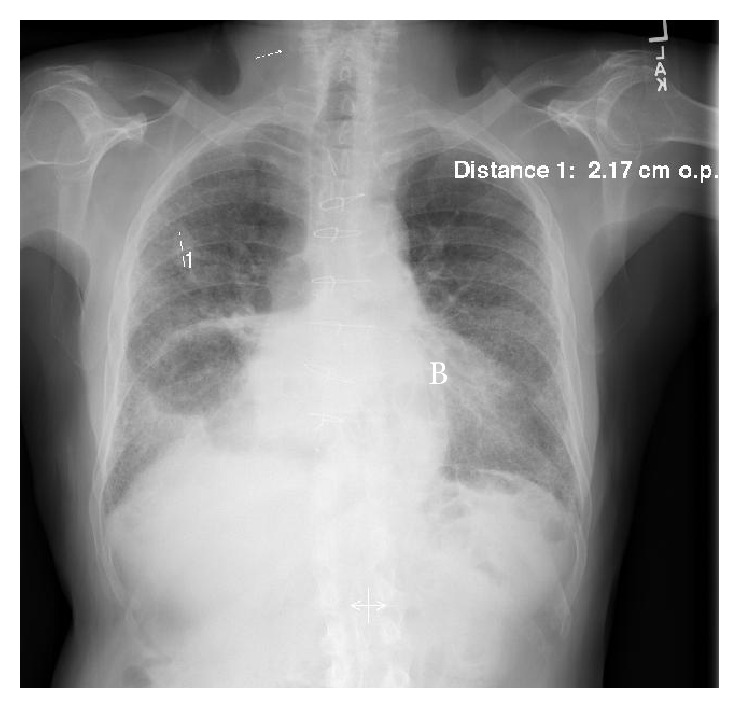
AP chest radiograph showing extensive bilateral reticular-fibrotic pattern with honeycombing and a 2.17 cm nodule in right upper lobe of his lungs.
